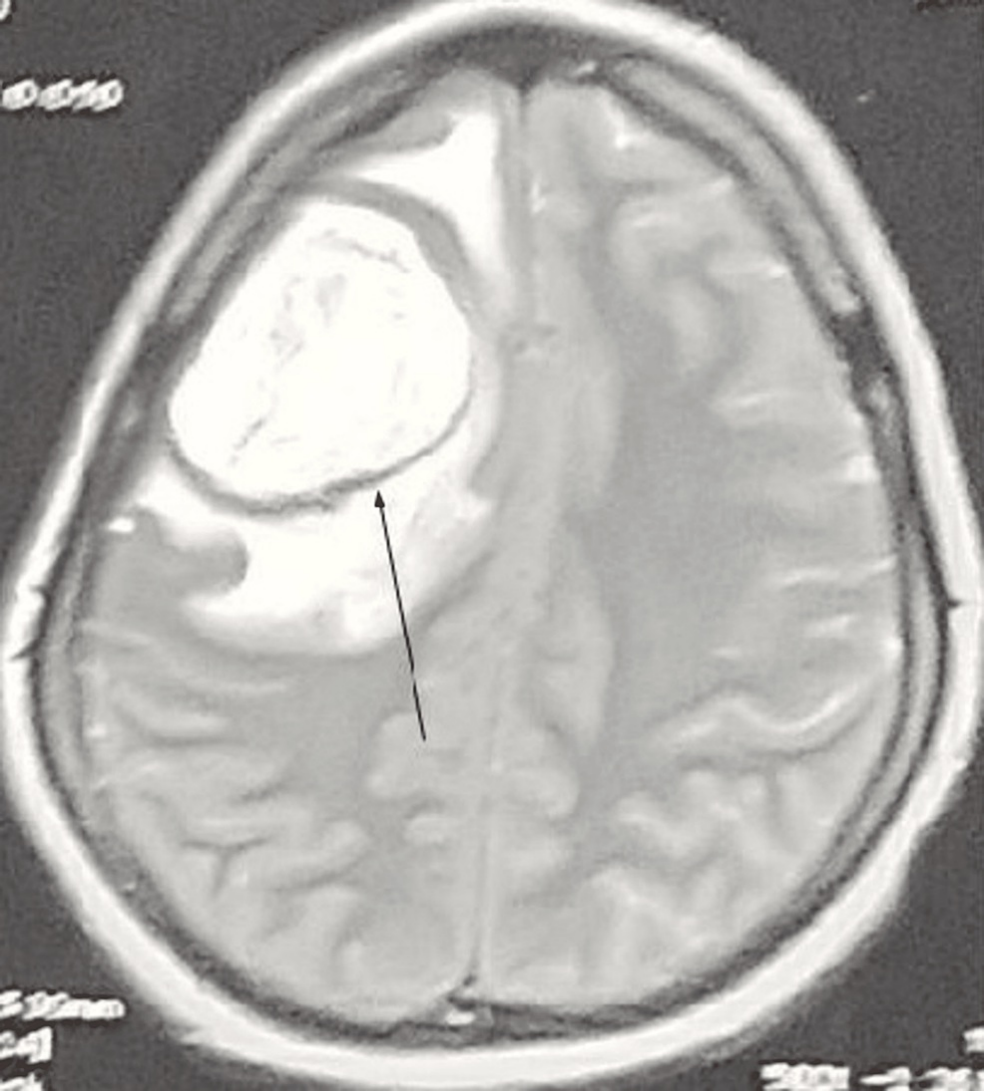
Axial MRI of the brain (T2-weighted sequence) showing enhancing hyperdense mass lesion in the right frontal lobe (arrow).
